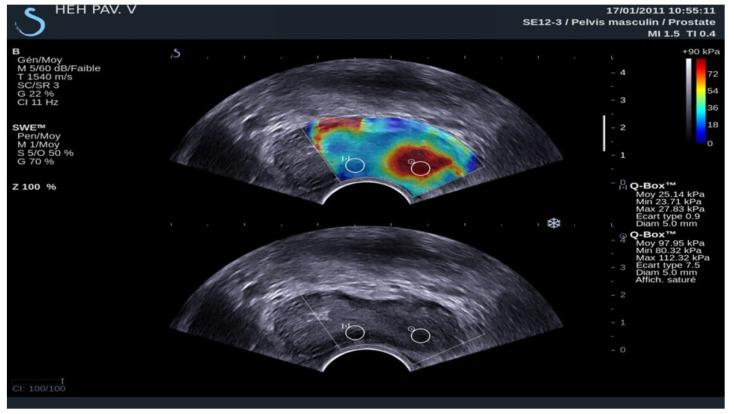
A 65-year-old patient with elevated PSA without other clinical data of interest. Image obtained using a SuperSonic Aixplorer ultrasound scanner with a multifrequency transrectal probe, bandwidth 3–12 MHz. Prostate cancer: the image identifies that the ROI of the left lobe has a mean hardness of 97.95 kPa, which is highly suspicious of malignancy. This finding was subsequently confirmed by biopsy.
